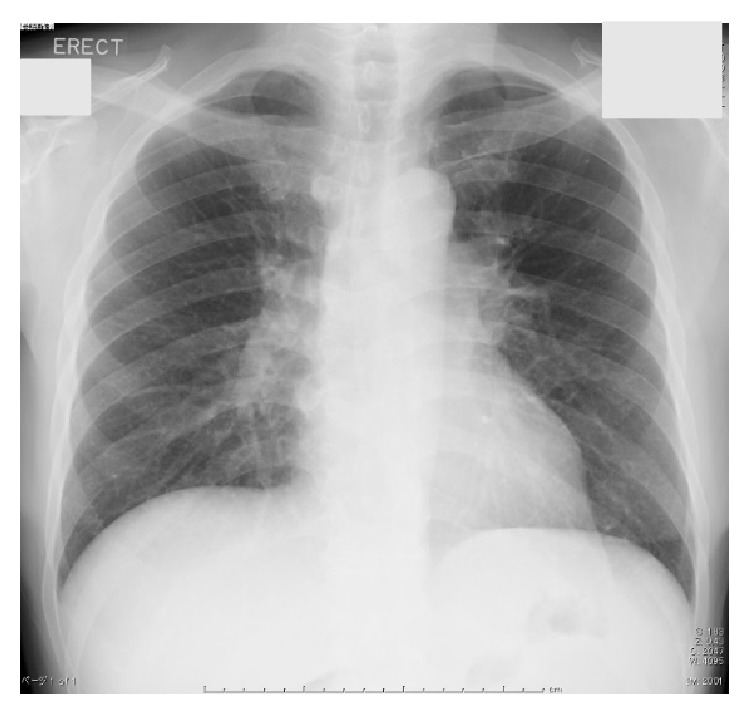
Chest radiography. Reticular and nodular shadows in both lower lobes of the lungs and enlargement of the pulmonary hilum.
